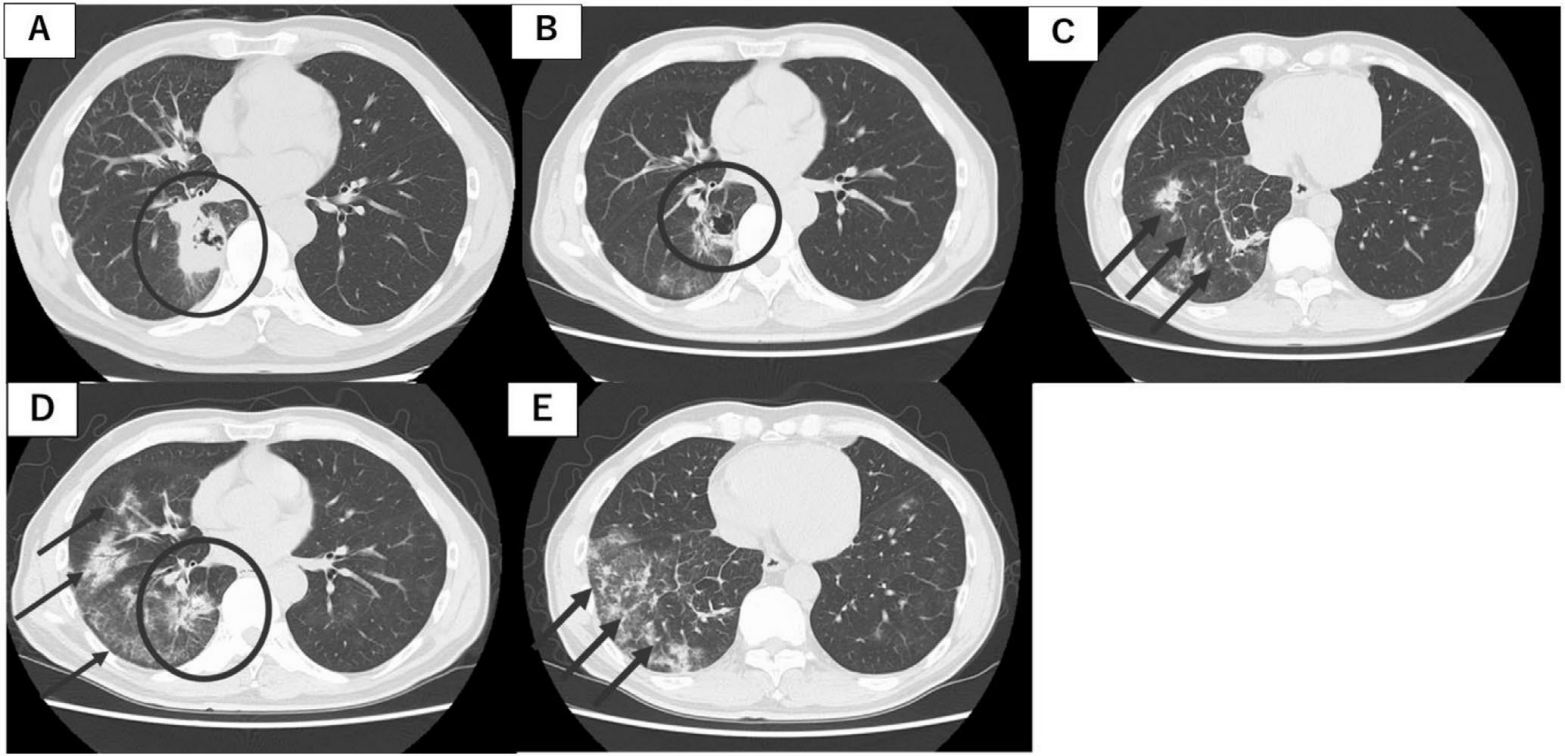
(A) Computed tomography (CT) showed a primary tumor in the S6 of the right lung (circle). (B) CT performed on Day 49. The primary lung tumor had shrunk (circle). (C) New ground‐glass opacity and consolidation appeared around the tumor (arrow). (D) CT showed the size of the primary tumor remained unchanged (circle). (D, E) ground‐glass opacity shadow developed (arrow).
